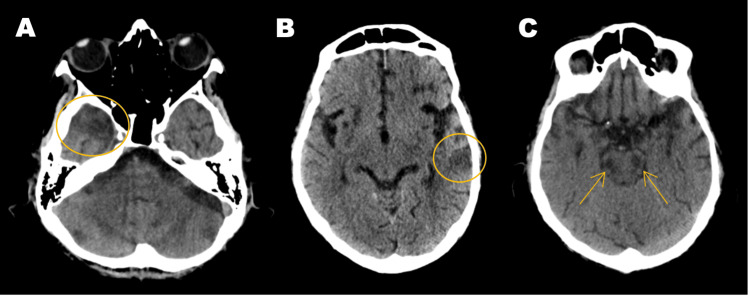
Brain CT scan A-C: In the follow-up CT scan, new areas of hypodensity were seen in the temporal lobes bilaterally (circles in A and B) and in the brainstem (arrows in C).
